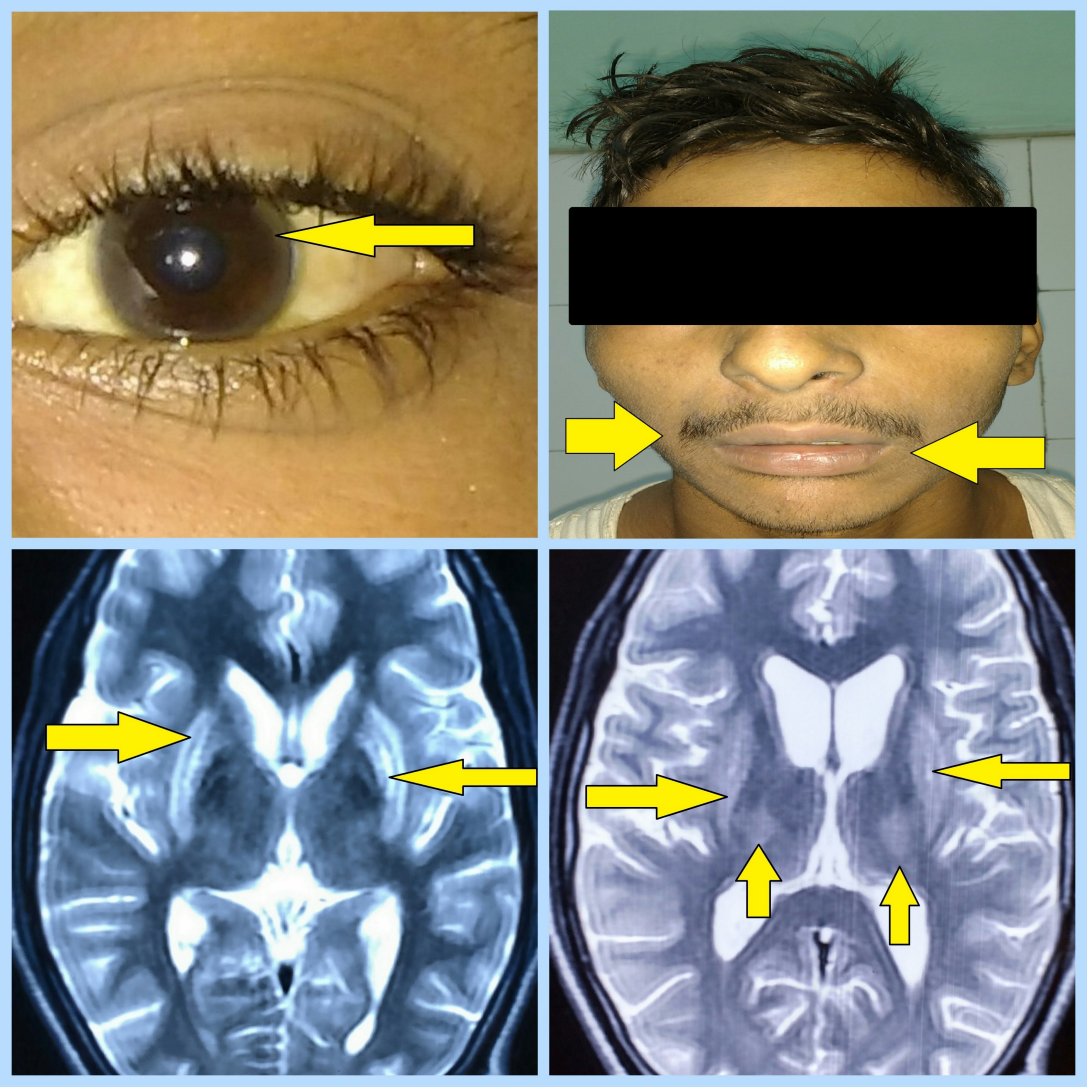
Figure Showing Kayser-Fleischer Rings, Mask-Like Facies, and Hyperintensity of Basal Ganglia and Surrounding Brain Gray Matter on MRI
